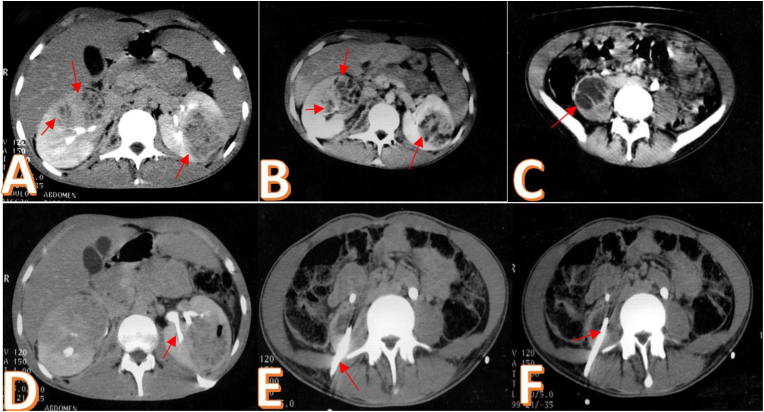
Computed tomography: A, Hypodense septate formations of both kidneys. C. Abscessed collection of the right psoas muscle. D. Drain in the left renal collection. E, F. Drain at the level of the right psoas collection.
